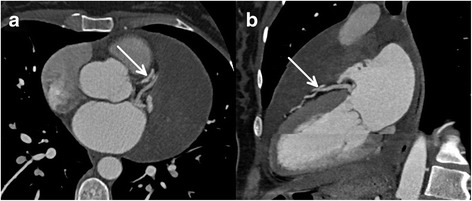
Coronary CTA images (panel a: axial plane, panel b: two-chamber view reconstruction) showed that coronary arteries were not invaded by the tumour. *Arrows* show the left anterior descending artery
